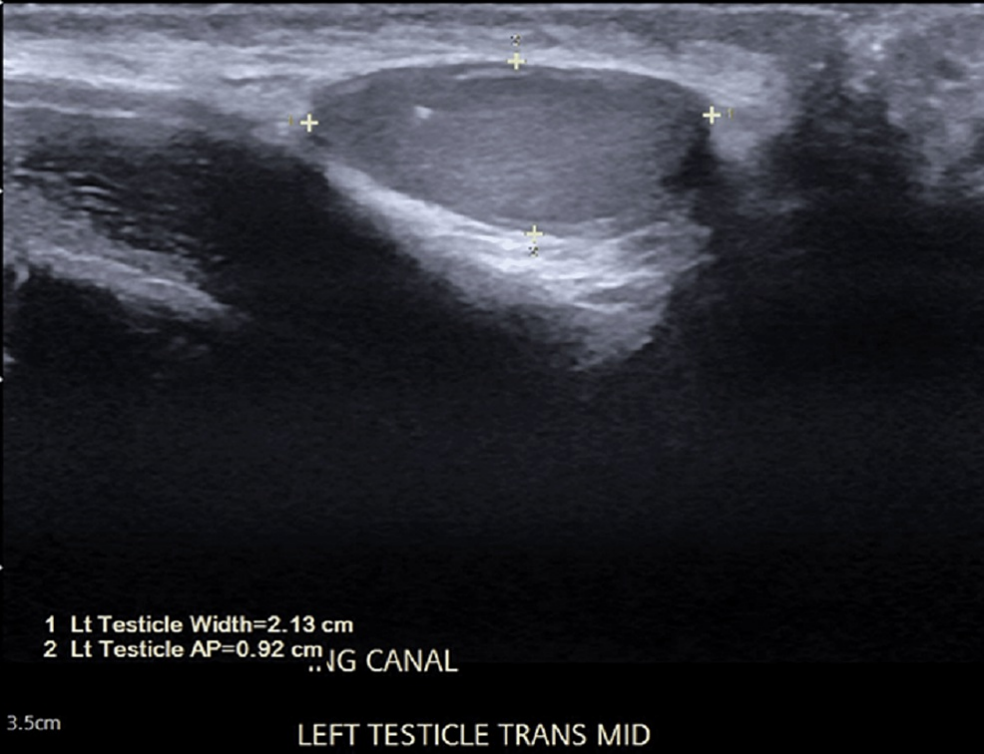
Pre-operative ultrasound of the patient in case 1 Ultrasound of the patient in case 1 two years ago, which demonstrated an asymmetric small size and mildly hypoechoic left testicle; the right testicle was descended and normal.
